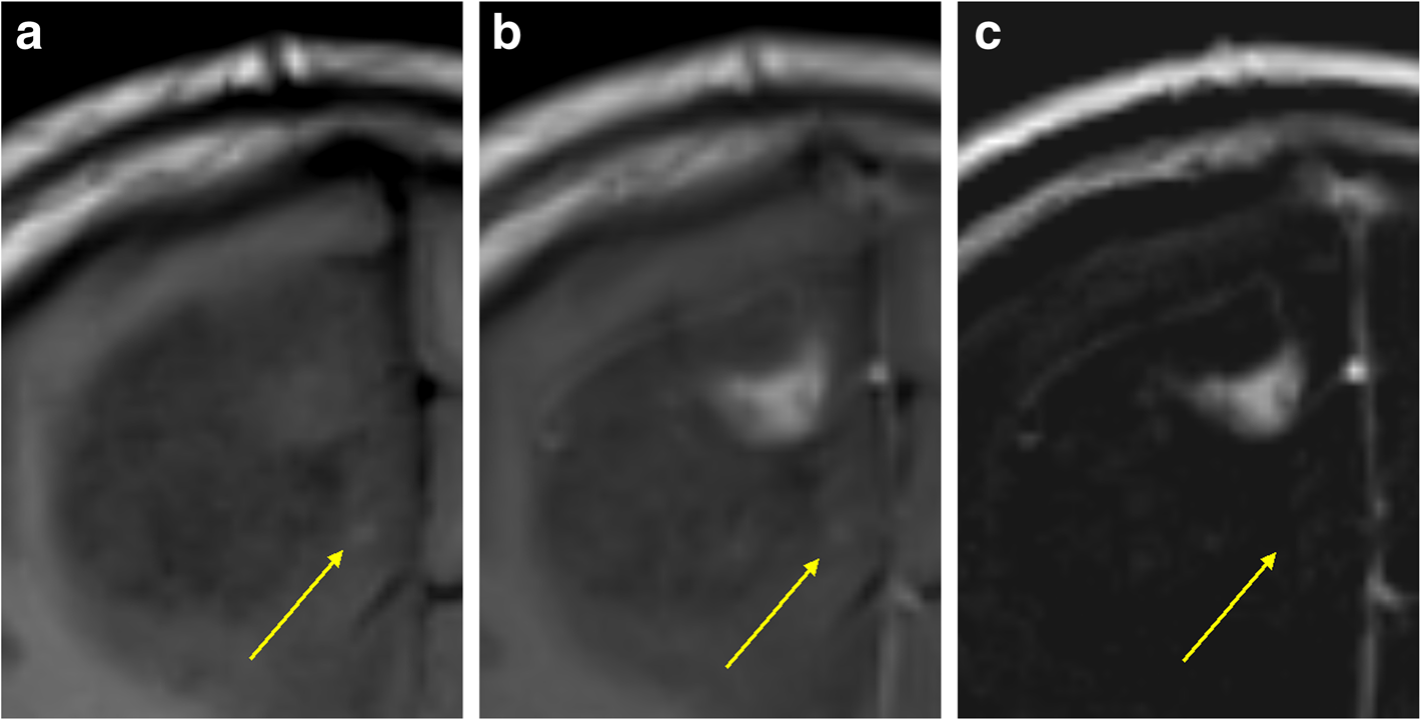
The quantitative enhancement (QE) analysis system considers both unenhanced (a) and contrast-enhanced (b) T1-weighted images when calculating the QE map (c) and thus knows that regions that are bright on the contrast-enhanced image (arrow) are not enhancing if they are equivalently bright in the unenhanced image
